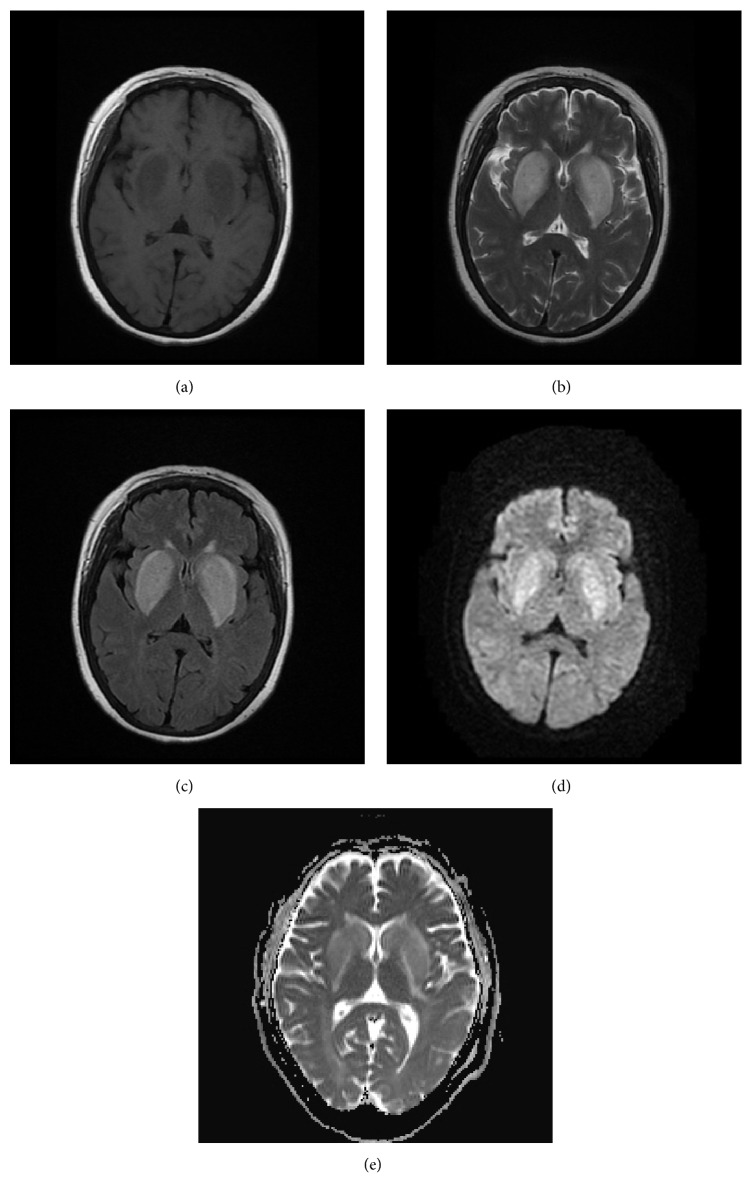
(a) Axial brain MRI showed symmetric hypointensity in the bilateral basal ganglia on T1-weighted images. (b, c) Axial brain MRI showed symmetric hyperintensity in the focal region on T2-weighted images and FLAIR images. (d) Diffusion-weighted imaging revealed slightly higher inhomogeneous signals in the involved regions. (e) ADC map revealed that the ADC value of the involved regions is not low.
